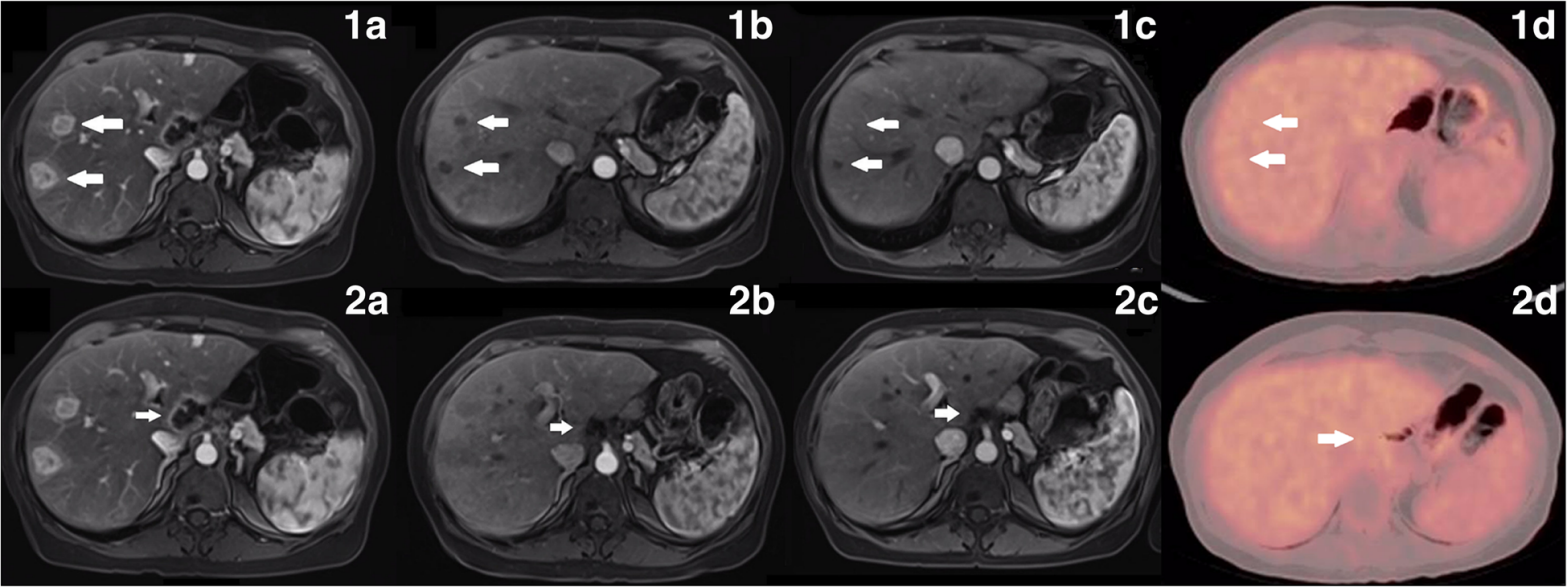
Columns a, b and c are magnetic resonance imaging and column d is a PET/CT imaging. White arrows in the first line show the reduction and complete remission of hepatic nodules. White arrows in the second line show the reduction and complete remission of a perihepatic lesion. a: Baseline images. b: Images after four treatment cycles. c: Images after eleven treatment cycles. d: Images after sixteen cycles
